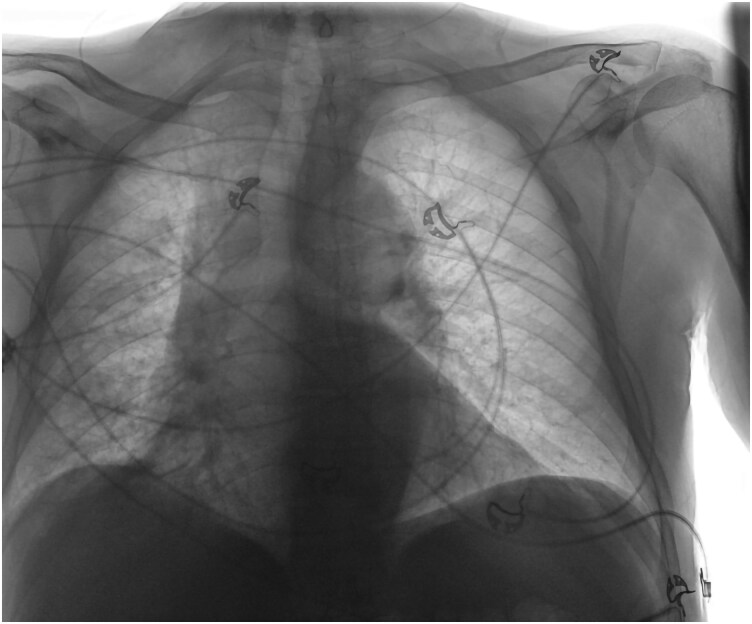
Chest radiograph at time of presentation demonstrated findings suggestive of pulmonary congestion, with an area suspicious for an underlying infiltrative process in the right middle lobe.
